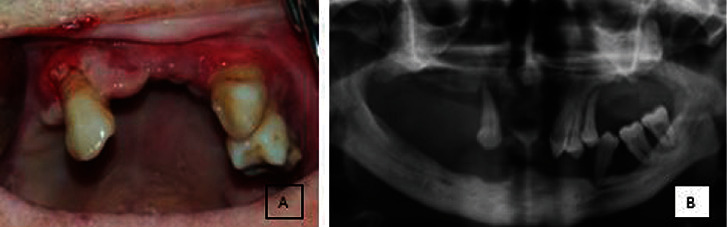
(a) The image showing that the clinical attachment loss was severe in the periodontal tissue. Bleeding, swelling, atrophy, and floppy were observed in the gingival tissue. (b) The panoramic radiography image of the patient.
